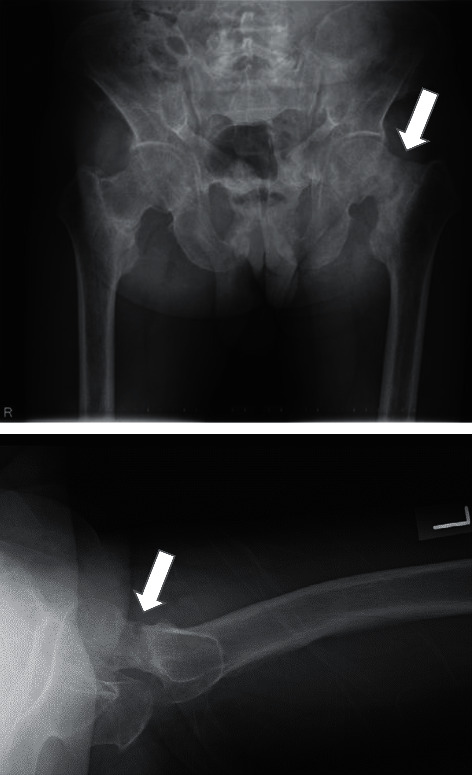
Radiographs in 2016 revealing left femoral neck fracture and varus deformity at the fracture site.
